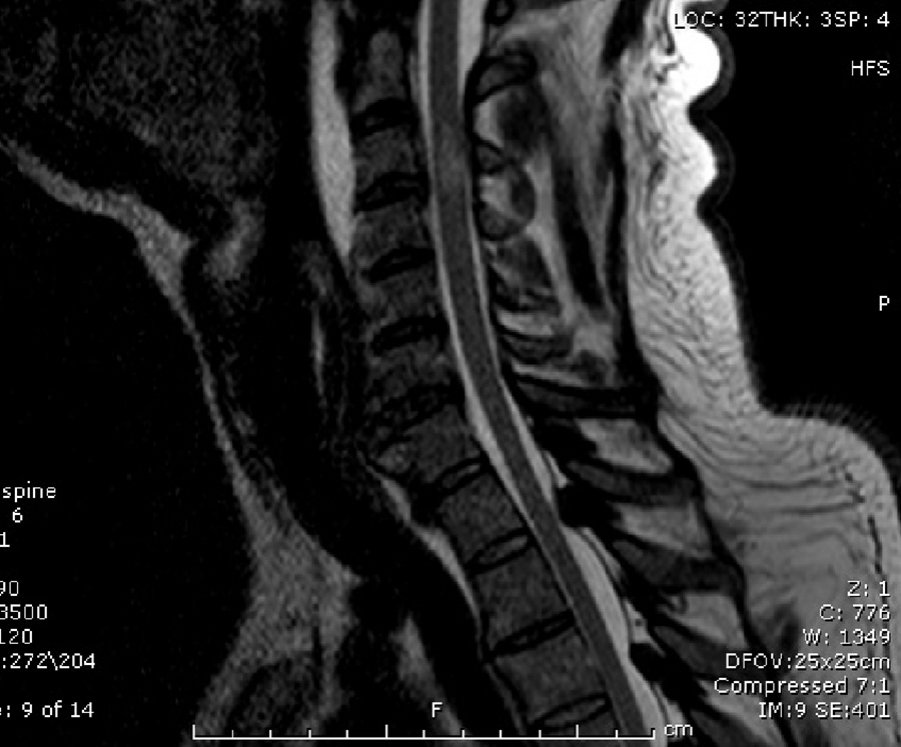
Preoperative sagittal cervical spine T2 MRI Cervical spine stenosis with a focal cord hyperintensity at the level of C3
